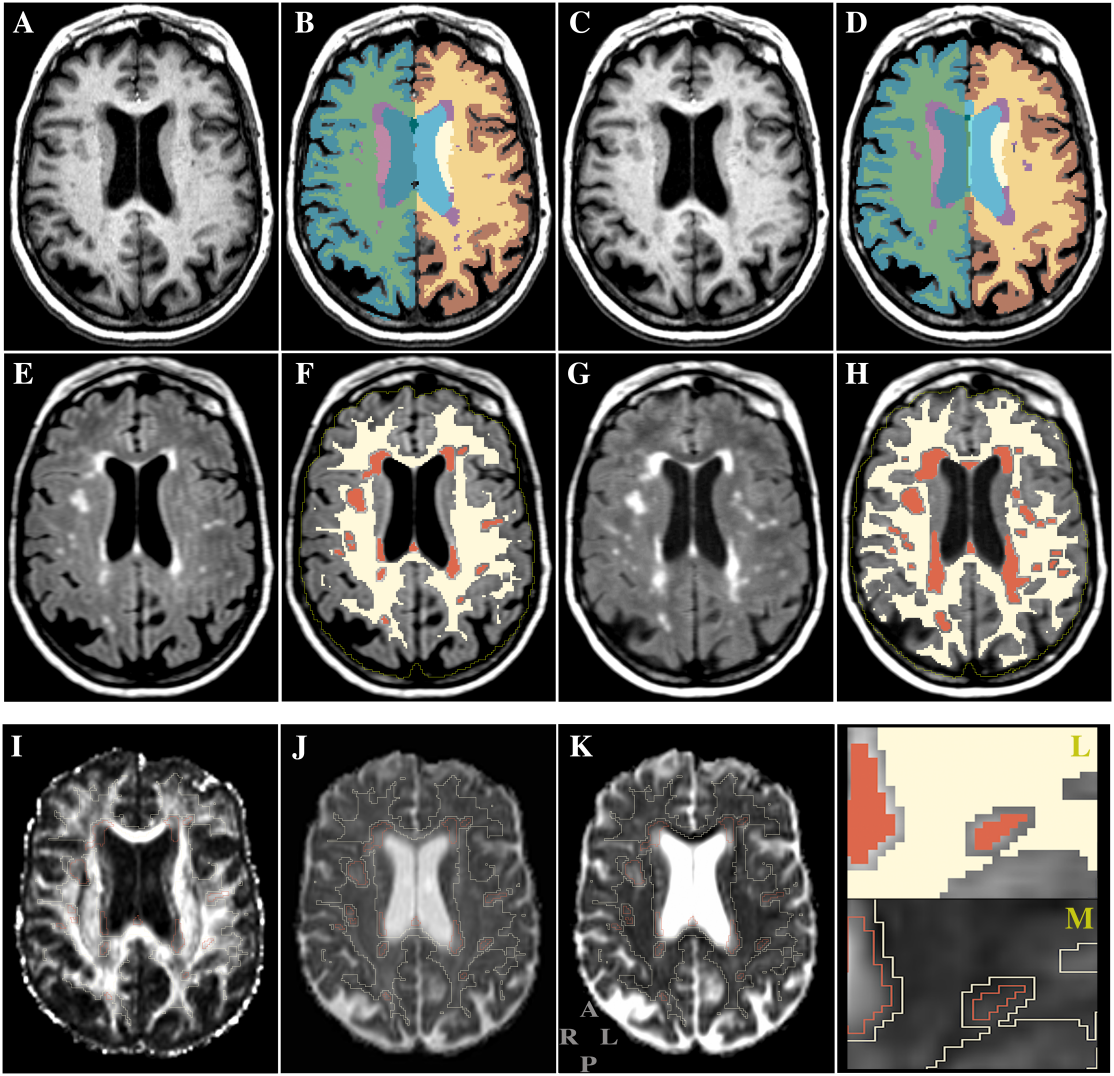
Example of structural and diffusion MR images from the study. Panels show the same brain region in axial view at baseline (A-B, E-F, I-K) and at 4-years (C-D, G-H). *Top row*: T1-weighted MPRAGE images. *Middle row*: T2-weighted FLAIR images. Panels B and D show the brain tissue segmentation maps (parc) obtained with FreeSurfer [37]. The parc WM and the WMH maps were merged as shown in F (baseline) and H (4 years) where NAWM (eroded) is indicated in light yellow and WMH in red. We used these (i.e. F and H) as masks to measure the diffusion indices within the NAWM and WMH compartments. The yellow line in panels F and H represents the outline of the intracranial cavity. *Bottom row*: panels show an example of diffusion maps from the baseline time point. The outline-only of NAWM and WMH map in panel F is overlaid on the FA (I), AD (J) and RD (K) images. The two small panels at bottom right show a close view of a section of the overlaid mask as filled (L, from panel F) and outlined (M, from panel K). Standard radiological orientation is shown at bottom left of panel K with A = anterior; R = right; L = left; P = posterior.
